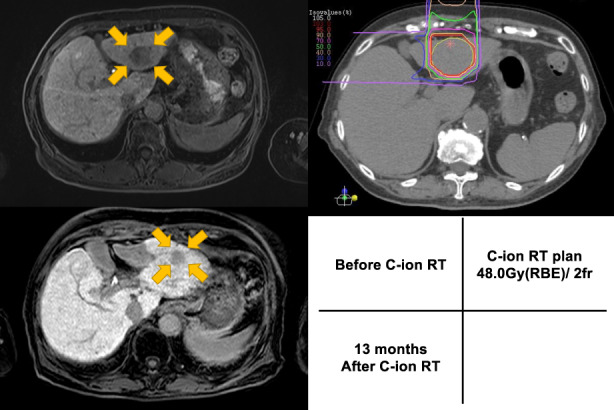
Case presentation: hepatocellular carcinoma of EOB‐MRI image before carbon‐ion radiotherapy, dose distribution of carbon‐ion radiotherapy, and EOB‐MRI image 13 months after treatment are shown.
